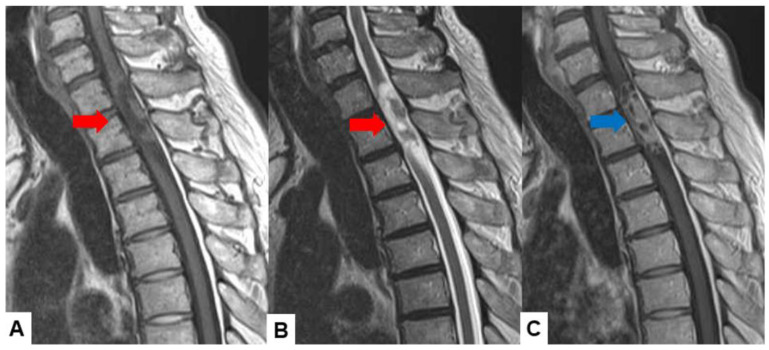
Fifty-four M, spinal schwannoma. (A) T1-weighted midsagittal image, (B) T2-weighted midsagittal image, and (C) enhanced T1-weighted midsagittal image. Red arrows indicate tumor and blue arrow shows tumor enhancement. The tumor is mixed-intensity because of tumor necrosis.
